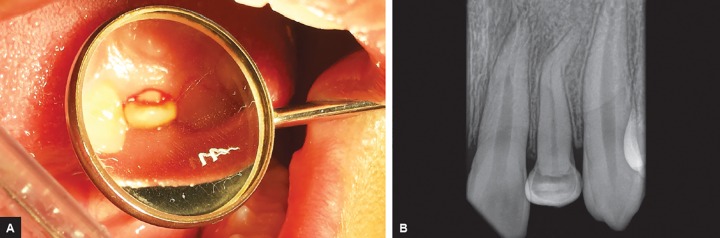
(A) Incremental reduction of palatal surface without any pulpal exposure, (B) periapical radiograph confirming the intact pulp chamber
